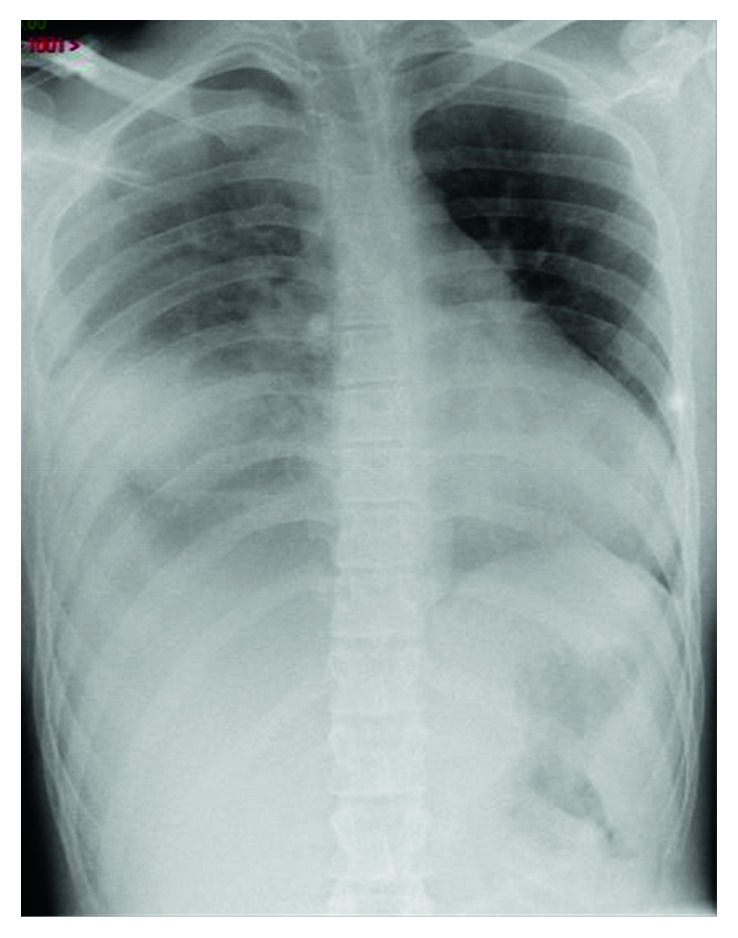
Thoracic radiograph showing the right hemopneumothorax.
